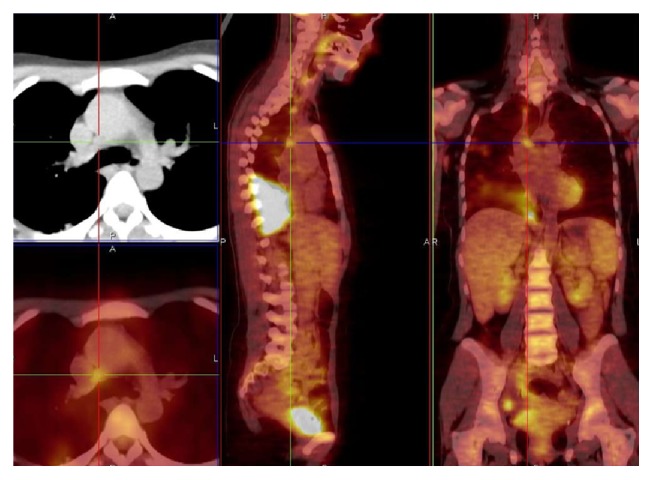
M. tuberculosis* infection with lymph node of Barety space (4R), SUV max 3.50. Related to patient 11 (PET/CT. Courtesy “V. Monaldi” Hospital).
